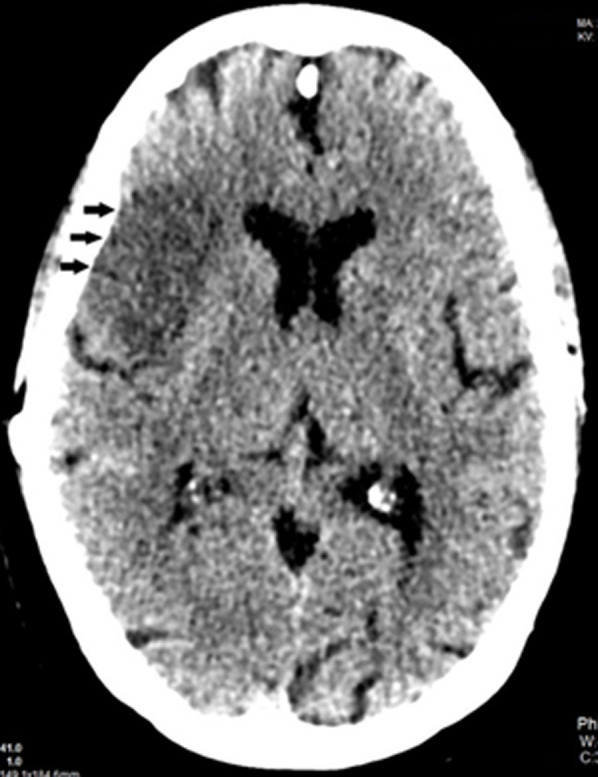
repeated brain computed tomography scan showed right middle cerebral artery territory infarct (black arrows) with no evidence of hemorrhagic transformation
